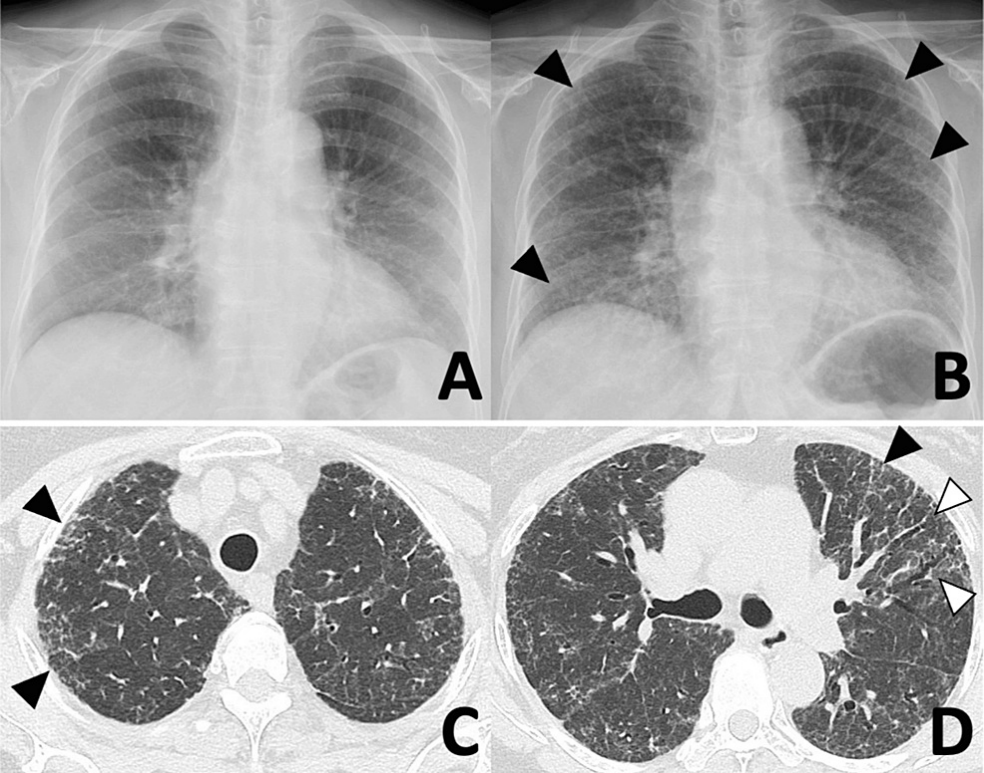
Chest radiograph and high-resolution CT of the chest Before the fifth BNT162b2 mRNA vaccination, a chest radiograph showed no abnormalities (A). After vaccination, infiltrative shadows (black arrows) were detected in the lower field of both lungs (B). Chest computed tomography revealed bilateral ground-glass opacities (black arrows), thickened interlobular septal walls, and traction bronchiectasis (white arrows) (C, D).
